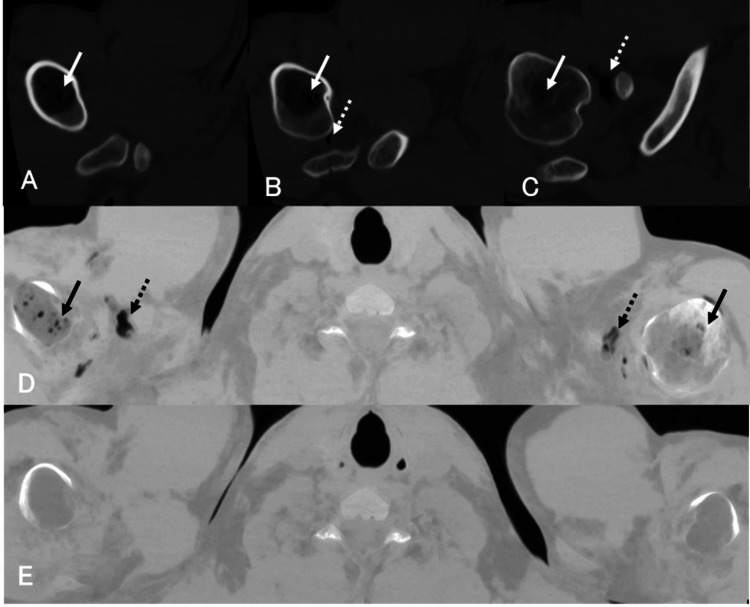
Case 1 A-C: Bone window settings. All computed tomography (CT) images were reviewed using a bone window setting with a window width of 3000 HU and a window level of 400 HU. D-E: Minimum intensity projection (MIP) images. Pre-hyperbaric oxygen therapy (HBOT) plain CT images (A-D) demonstrate multiple gas densities in the proximal humeral bone marrow bilaterally (solid arrows) and the right shoulder joint (dashed arrows). E: Complete resolution of intramedullary gas following HBOT.
